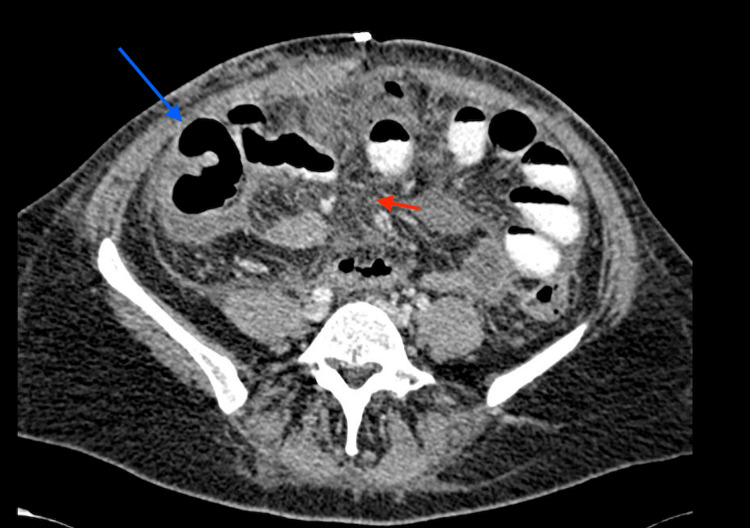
Repeat CT scan after initial surgery showing dilated bowel loops (blue arrow) and mesenteric fat stranding (red arrow). No evidence of a contrast leak.
